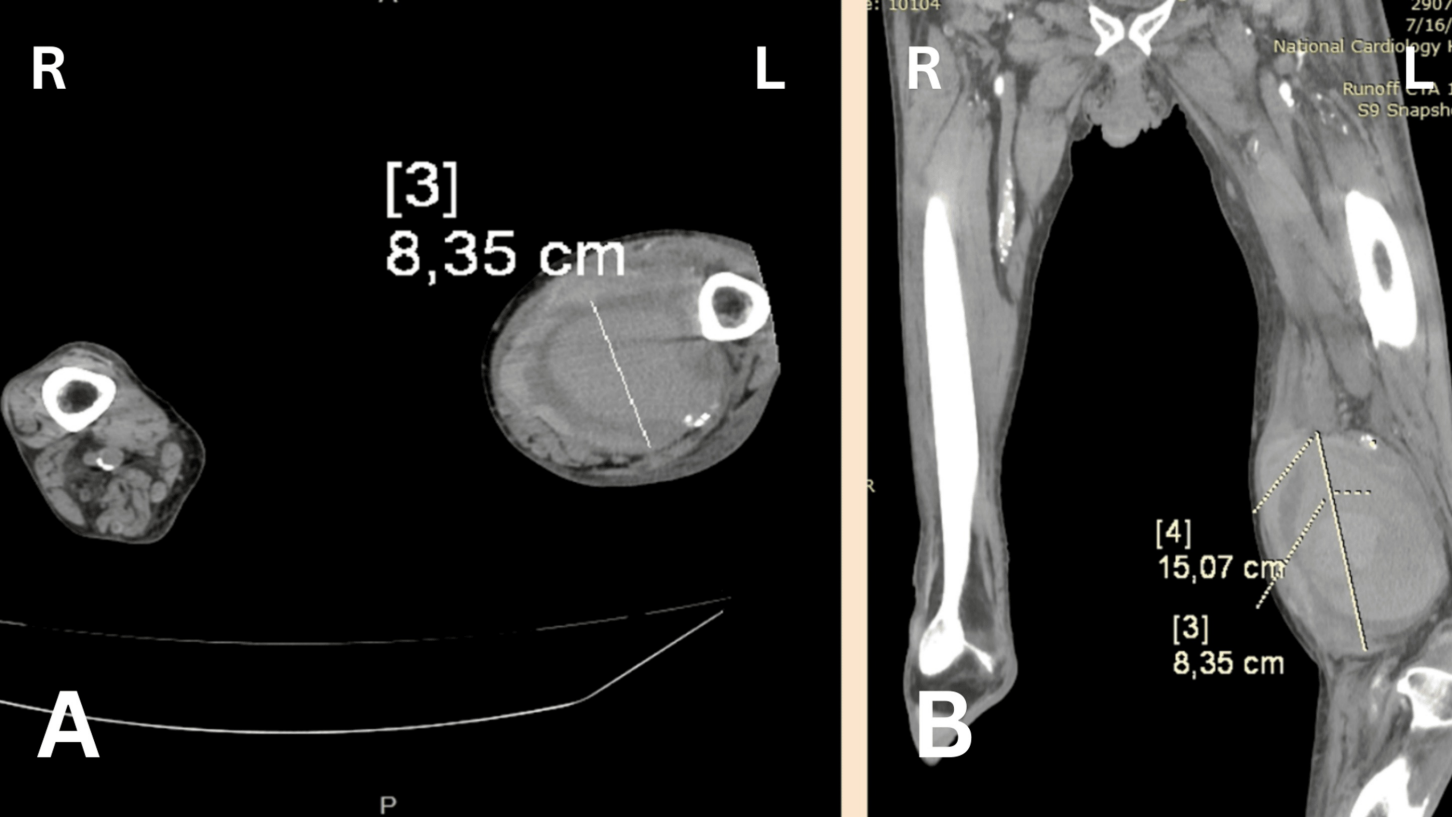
CT angiography of the popliteal artery pseudoaneurysm. A: Transversal view. B: Sagittal view.
